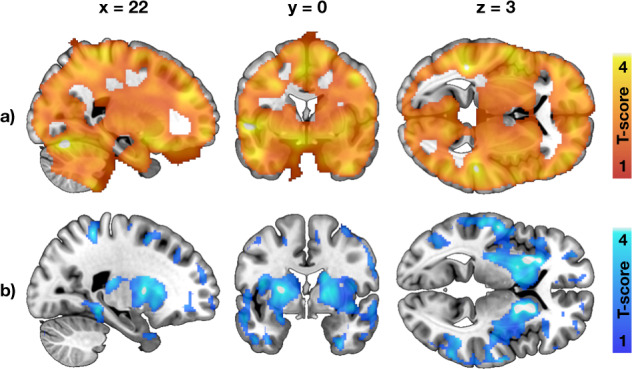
Effects of familial obesity risk to central glucose uptake and μ-opioid receptor availability. a Brain regions where higher Family Risk score associated with increased brain glucose uptake in the 38 individuals studied with [^18^F]FDG. b Brain regions where higher Family Risk score associated with lower μ-opioid receptor availability in the 41 individuals studied with [^11^C]carfentanil. The effects of familial obesity risk we global for brain glucose uptake, whereas the associations were most prominent in striatum and insula for μ-opioid receptors. The images show results from SnPM13 linear regression, with age and other risk factors (BMI, physical exercise) as covariates. The data are thresholded at *p* < 0.05, FWE corrected at cluster level.
